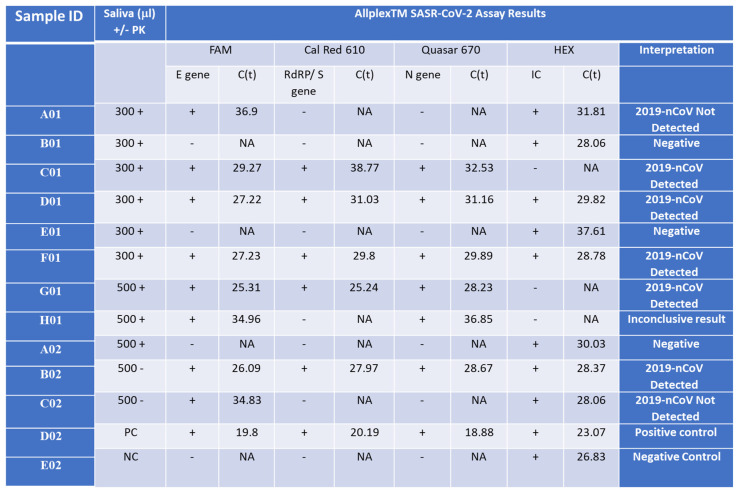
Detection of the Wuhan SARS-CoV-2 in saliva samples from infected patients. Saliva samples (300 or 500 µL) were collected from SARS-CoV-2 patients with Ct lower than 34 for the E gene (C01, D01, F01, G01 = B02), a SARS-CoV-2 patient with Ct higher than 34 or the E gene (A01 = H01 = C02), and control patients who represent normal and symptomatic flu-like patients who were negative with the Seegene test (B01, E01, A02) and incubated with (+PK) or without (-PK) proteinase K. D02 and E02 are positive and negative control (PC and NC), respectively, provided by the Seegen Allplex TM assay. The Seegene gold-standard protocol involves four different primer sets, each targeting specific regions (E, S, and N genes) of the SARS-CoV-2 viral genome, along with a fourth primer set for a spiked positive control sample (HEX). A qPCR assay with no template was used as a negative control (NC) for the qPCR assay. The interpretation column indicates the call by the gold-standard Seegene platform. ‘NA’ stands for ‘not applicable’, indicating no detected CT value. ‘2019-nCoV Detected’ denotes the declaration of a SARS-CoV-2-positive sample. ‘Negative’ indicates a clear-cut negative sample. ‘2019-nCoV Not Detected’ refers to a borderline sample. ‘Positive control’ denotes an internal positive control amplicon sample provided by the manufacturer, while ‘Negative control’ indicates no template.
